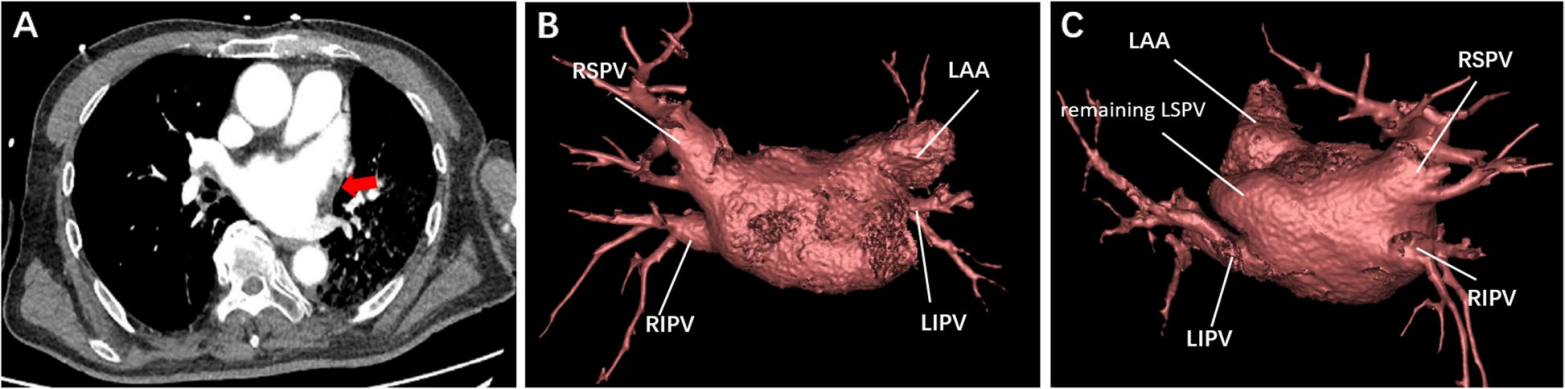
Absence of LSPV branches on postoperative chest CTA. A Filling defect in LSPV branches on CTA (red arrow). B Absence of LSPV on three-dimensionally reconstructed chest CTA (anterior view of left atrium). C Remaining main trunk of LSPV on three-dimensionally reconstructed chest CTA (posterior view of left atrium). CTA = computed tomography angiography; RSPV = right superior pulmonary vein; RIPV = right inferior pulmonary vein; LSPV = left superior pulmonary vein; LIPV = left inferior pulmonary vein; LAA = left atrial appendage
